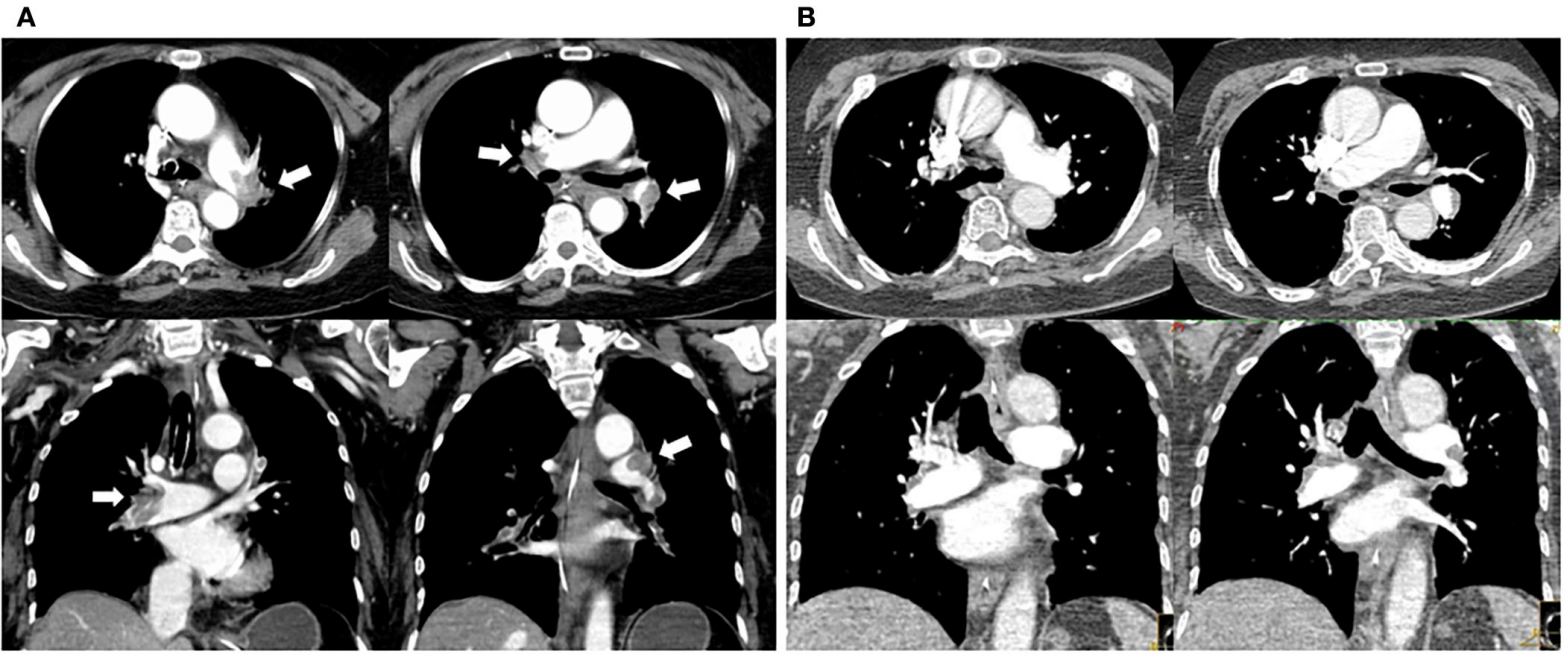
Changes in pulmonary embolism of CT pulmonary arteriography before and after percutaneous catheter-directed therapy. (A) Multiple filling defects in bilateral pulmonary artery trunks and their branches (white arrow) before CDT. (B) Thrombus eliminated 1 month after CDT and anticoagulation.
